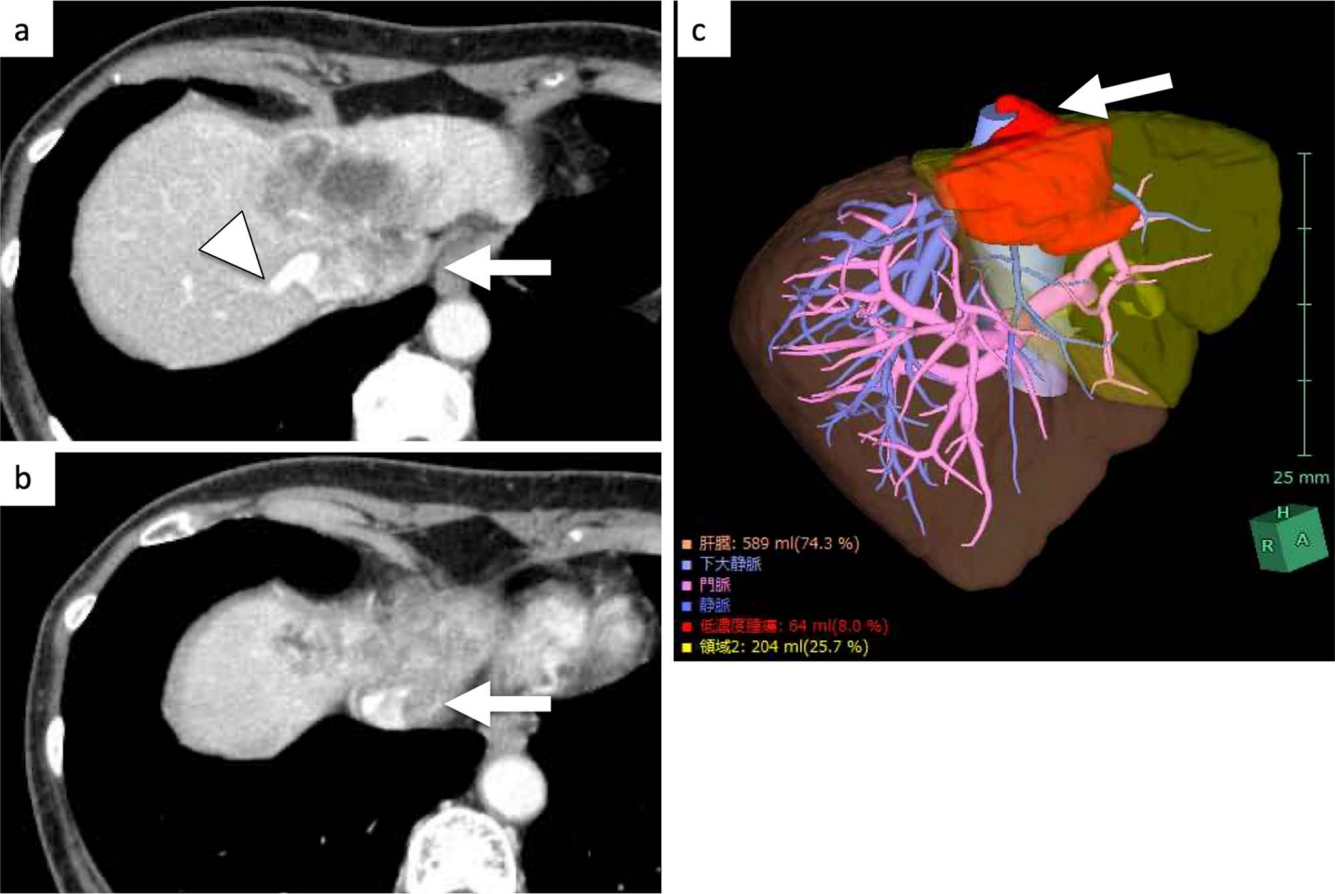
CT showed a mass-forming intrahepatic cholangiocarcinoma, 7 cm in diameter, in segment 4 (a). The ICC invaded the suprahepatic IVC, the MHV, and the LHV confluence (arrow, a–c), while the tumor did not invade the RHV (arrowhead, a)
